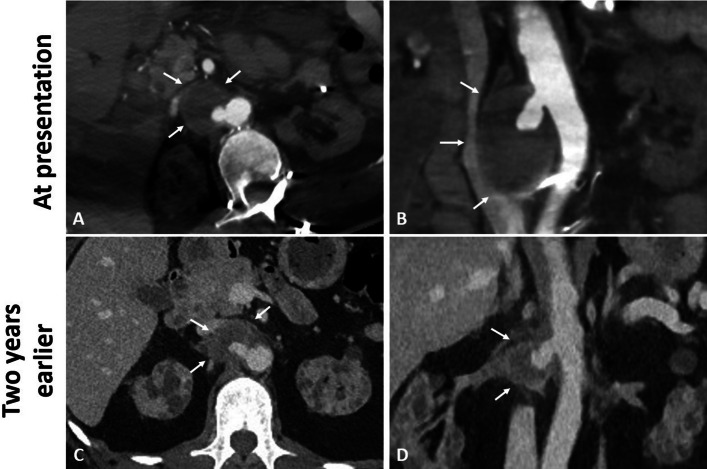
Aneurysm of the ostium of the right renal artery. Axial (A) and coronal (B) CT slices showing a 45 mm partially thrombosed aneurysm (arrows) located at the ostium of the right renal artery. The right renal artery was occluded downstream of the aneurysm. The aneurysm had a neck measuring 8 mm. Two years before, the aneurysm (arrows) measured 23 mm, as shown on axial (C) and coronal CT slices (D)
